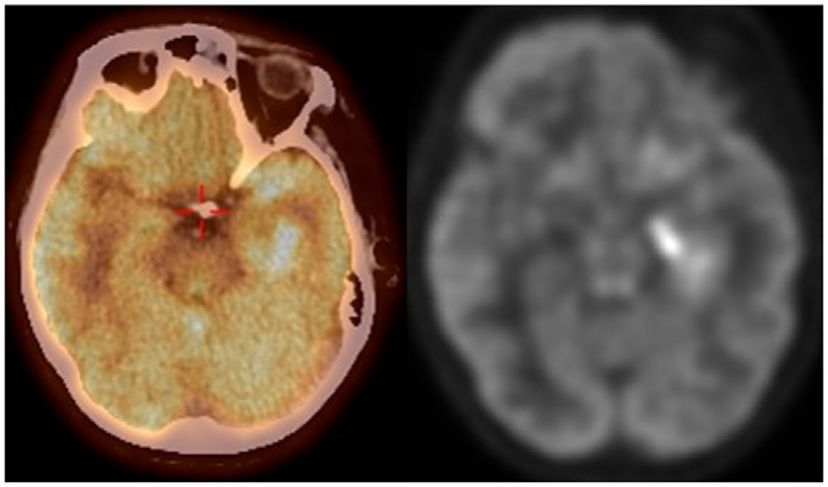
FDG PET CT scan demonstrating increased FDG uptake in the medial left temporal lobe corresponding to the area of increased FLAIR signal seen on MRI brain.
